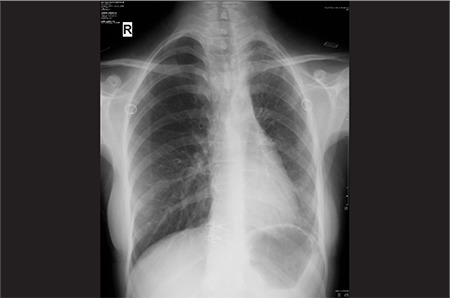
Chest radiograph shows small sized left lung and slight increase of interstitial markings on left. Right to left mediastinal shift and hypertrophic right lung is also seen
